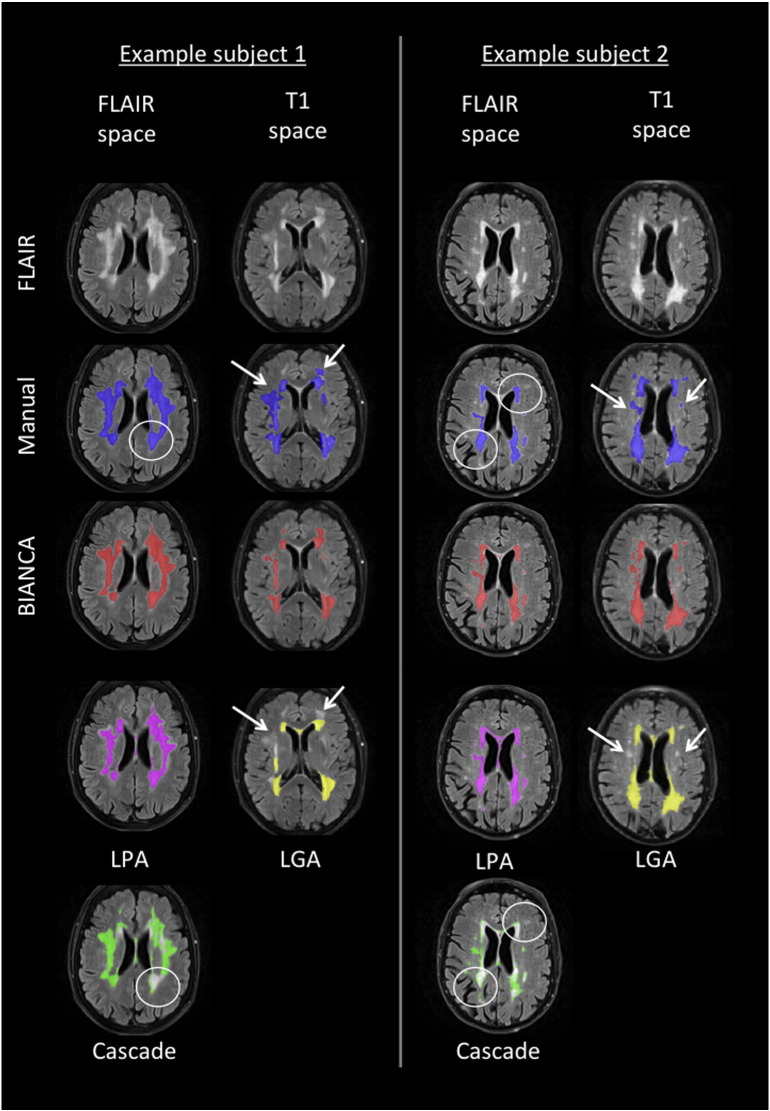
Example WMH segmentation with different tools. FLAIR images from two subjects are shown, together with the manual mask and the output from the different segmentation tools either in FLAIR space or T1w space, according to the reference space used for WMH segmentation by the algorithm. CASCADE (threshold = 0.8) and LPA gave the best performance on subject number one, while subject number 2 was the one giving the best LGA (kappa = 0.2) performance. White arrows and circles highlight the main segmentation errors by CASCADE and LGA, while BIANCA and LPA show good performance in both examples.
